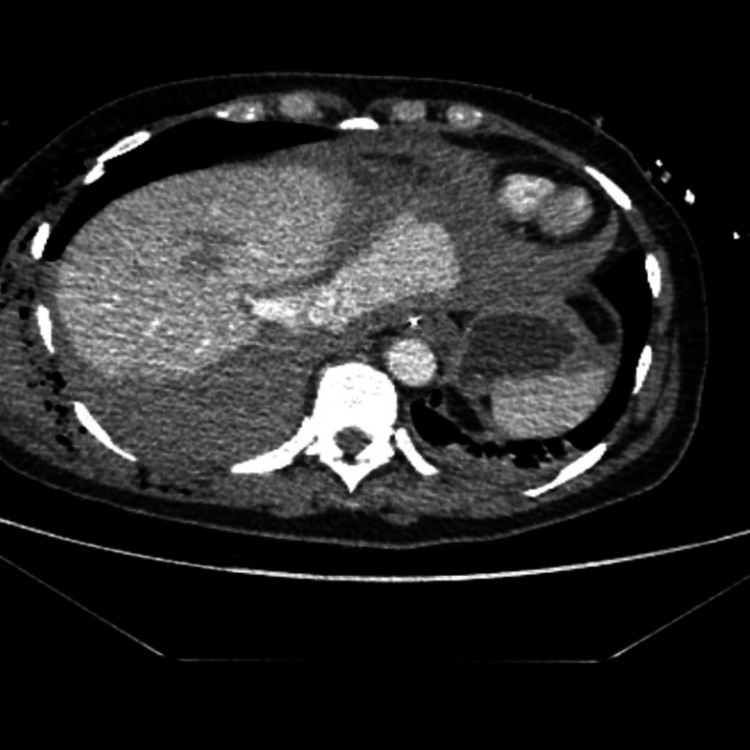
Radiological findings Axial images of CT demonstrating the active bleeding from the suprahepatic IVC injury CT: computed tomography; IVC: inferior vena cava
